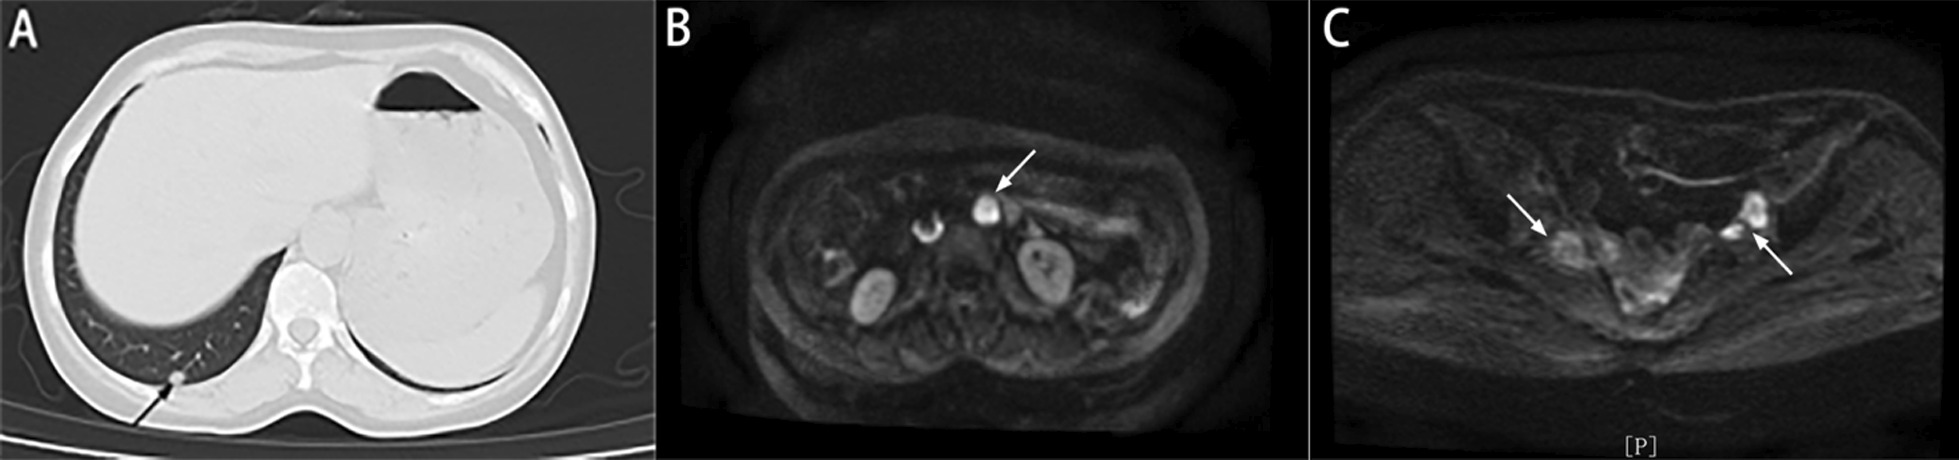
Imaging data of September 2021 A CT showed metastatic nodules in the right lung (arrow). B MRI showed enlarged lymph nodes in the retroperitoneum (arrow). C The pelvic nodules exhibited no obvious change (arrow)
